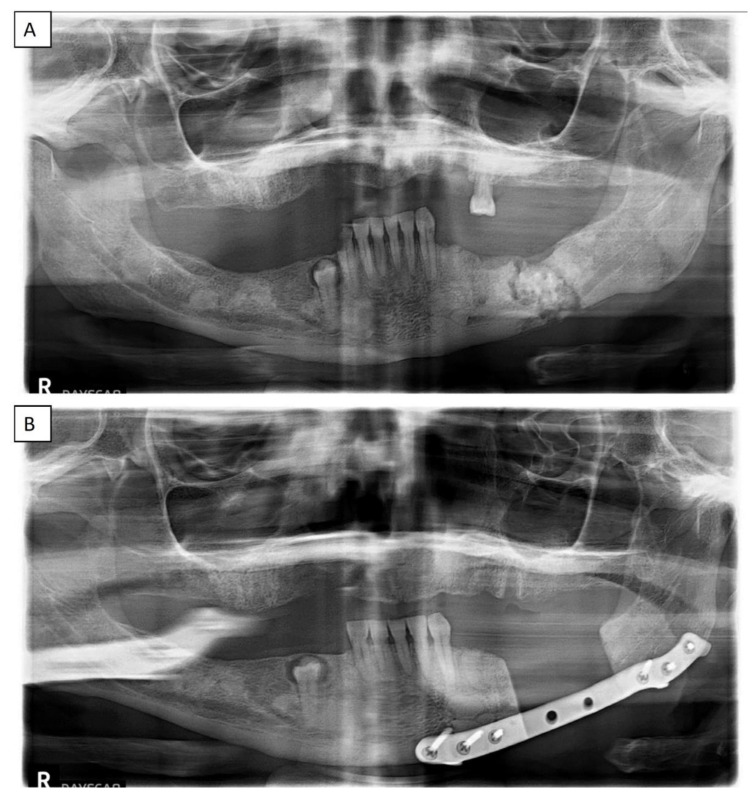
Florid cemento-osseous dysplasia involving both quadrants of the mandible in a symptomatic 63-year-old African American female (A). Post-resection and reconstruction radiograph (B).
